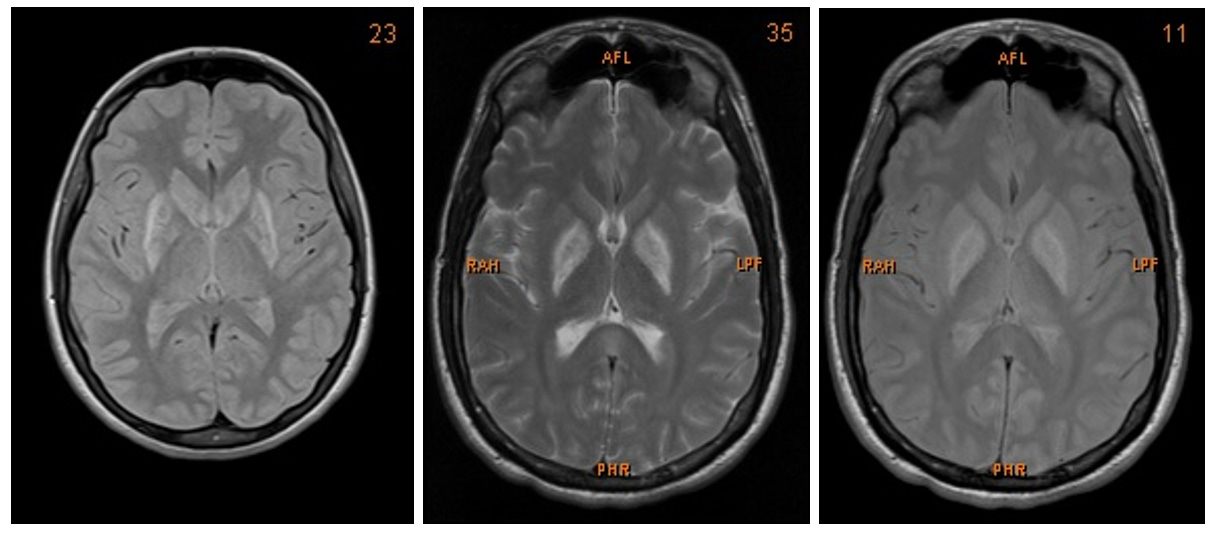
MRI images showing bilateral post-ischaemic lesions of the basal ganglia.
